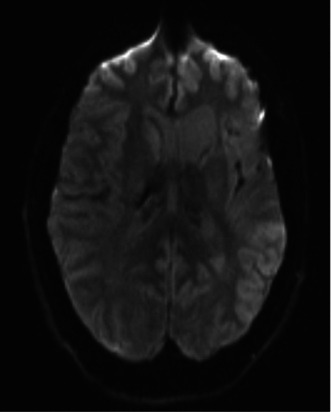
FIGURE 1 MRI brain demonstrated diffusion restriction in the bilateral basal ganglia with multifocal cortical ribboning, more prominent on the left.
